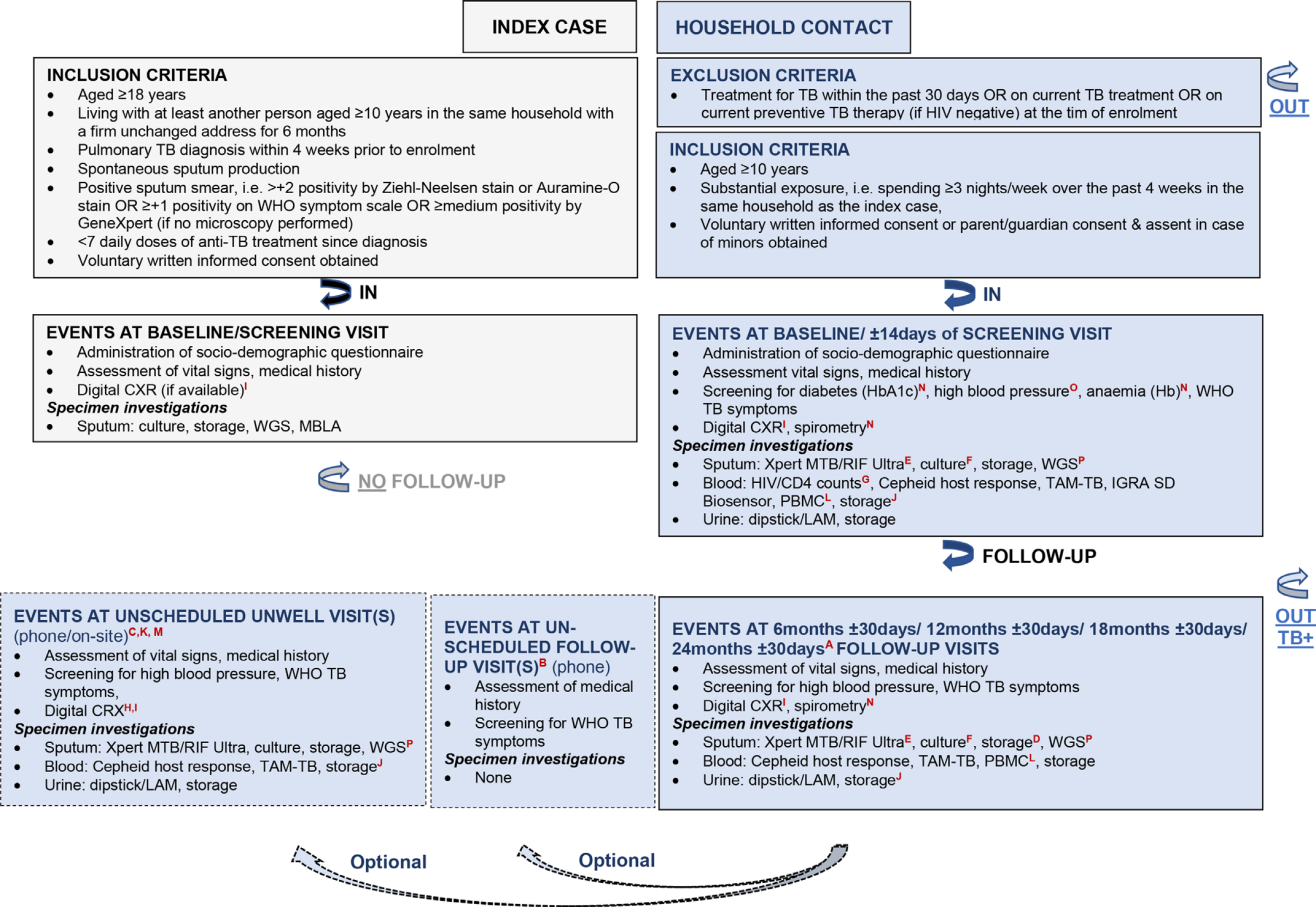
Eligibility criteria and schedules of events for index cases and household contacts. A=depending on the time point of study enrolment and consequently on the duration available for follow-up, that is, 18 or 24 months, the follow-up visit at 24 months±30 days may be conditional; B=the follow-up visit by phone may be conducted after the last scheduled follow-up visit at 18 months±30 days or 24 months ±30 days to assess whether symptoms suggestive of TB have occurred, TB diagnosis has been made or anti-TB treatment has been initiated; C=unwell visits by phone or on-site may be conducted between scheduled follow-up visits if a participant presents at a recruitment healthcare facility with signs and symptoms suggestive of TB; D=coached spontaneous or induced sputum collection for storage at scheduled follow-up visit at 18 months±30 days or 24 months ±30 days, and for repetition of HIV testing if tested negative at baseline; E=coached spontaneous or induced sputum collection on the decision of the investigating team for testing by Xpert MTB/RIF Ultra if participant presents with signs and symptoms suggestive of TB; F=coached spontaneous or induced sputum collection in case of Xpert MTB/RIF Ultra positivity or strong clinical suspicion of TB for repetition of the Xpert MTB/RIF Ultra; G=in case of HIV positivity to be followed by the assessment of CD4 counts; H=CXR to be conducted at an unscheduled on-site unwell visit on the decision of the investigating team depending on the nature of symptoms reported, and the time elapsed since the last CXR including its findings; I=not to be conducted among pregnant women; J=stored venous blood includes 6 mL EDTA blood for whole blood and plasma, 4 mL serum and 2.5 mL PAXgene blood, all samples will be deep frozen for retrospective testing using new diagnostics as described in text; K=in case the evaluation of symptoms of a participant unable to present at a recruitment healthcare facility is required an unscheduled on-site or home visit will be arranged by phone, the resolution of symptoms can alternatively be addressed by phone; L=collection of PBMC at baseline and follow-up visit at 6 months±30 days is optional, thus will not be performed at each participating site and for each participant; M=in case the evaluation of symptoms of a participant unable to present at a recruitment healthcare facility is required or doubtful if required an unscheduled unwell visit by phone will be arranged, the resolution of symptoms can alternatively be addressed by phone; N=spirometry and/or diabetes (HbA1c) will be performed at scheduled follow-up visits at 6 months±30 days, 12 months ±30 days and 18 or 24 months±30 days if required or not performed at baseline, anaemia (Hb) will be performed at baseline and scheduled follow-up visits at 6 months±30 days, 12 months ±30 days and 18 or 24 months±30 days if possible; O=blood pressure measurement will be performed at baseline and scheduled follow-up visits at 6 months±30 days, 12 months ±30 days and 18 or 24 months±30 days; P=WGS to be performed once *Mtb* infection is confirmed and an isolate could be recovered. CD4, cluster of differentiation 4; CXR, chest radiograph; Hb, haemoglobin; HbA1c, glycated haemoglobin; IGRA, interferon gamma release assay; LAM, lioparabinomannan; MTB, *Mycobacterium tuberculosis*; MBLA, molecular bacterial load assay; PBMC, peripheral blood mononuclear cell; RIF, rifampicin; TAM-TB, T- cell activation marker tuberculosis; TB, tuberculosis; WGS, whole genome sequencing.
